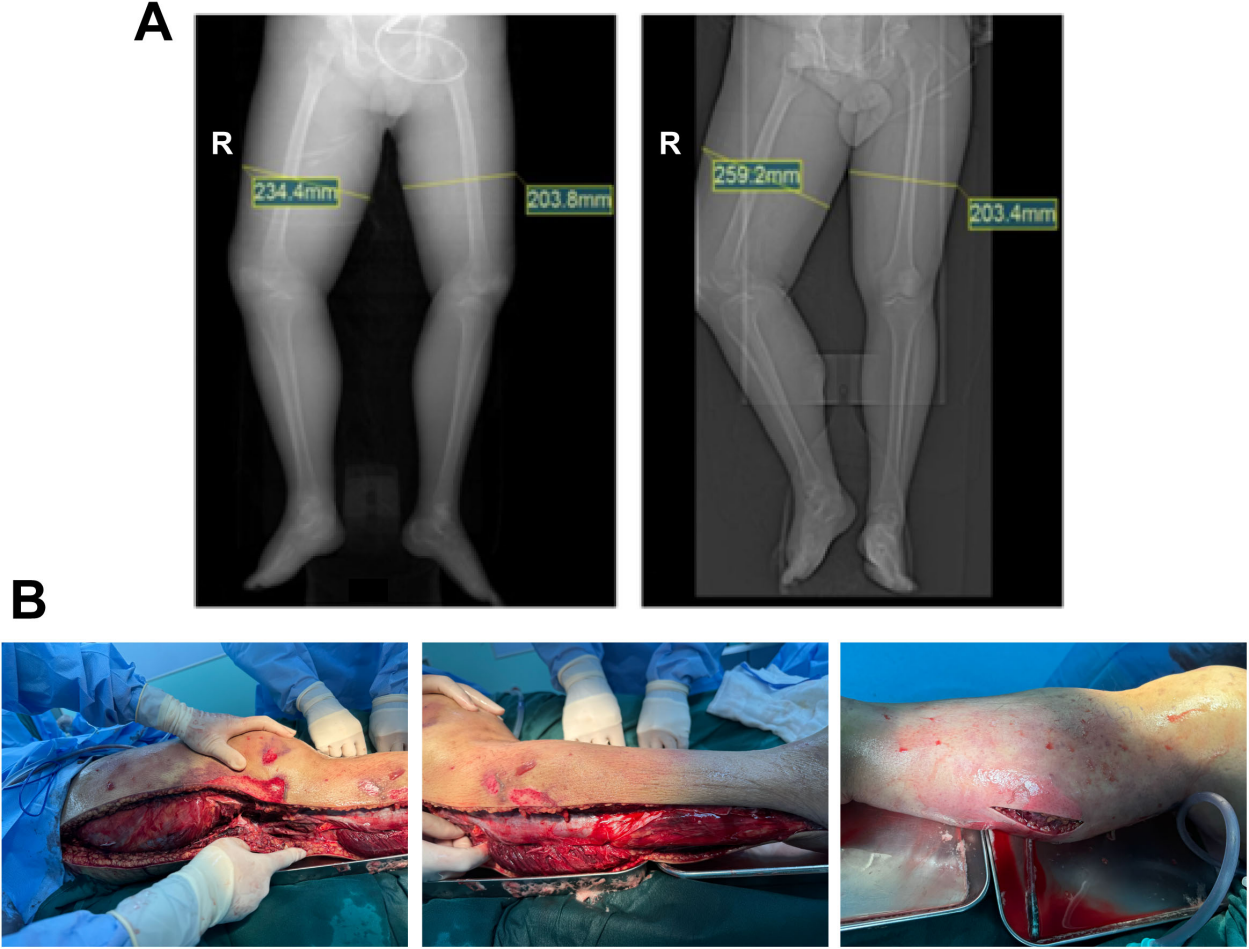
Preoperative imaging and intraoperative findings. (A, left) CT localization scan of both lower limbs at 02:17 on June 26, 2025, showed marked thickening of the right thigh soft tissue, measuring 234.4 mm (right thigh) and 203.8 mm (left thigh). (A, right) CT scan at 10:26 on June 26, 2025, indicated significant progression of right thigh soft tissue swelling, now measuring 259.2 mm (right thigh) and 203.4 mm (left thigh). (B) Intraoperative exploration revealed pronounced edema of the right lower limb fascia and areas of muscle that appeared gray-white and inelastic, consistent with necrotic changes and diagnostic for necrotizing fasciitis.
